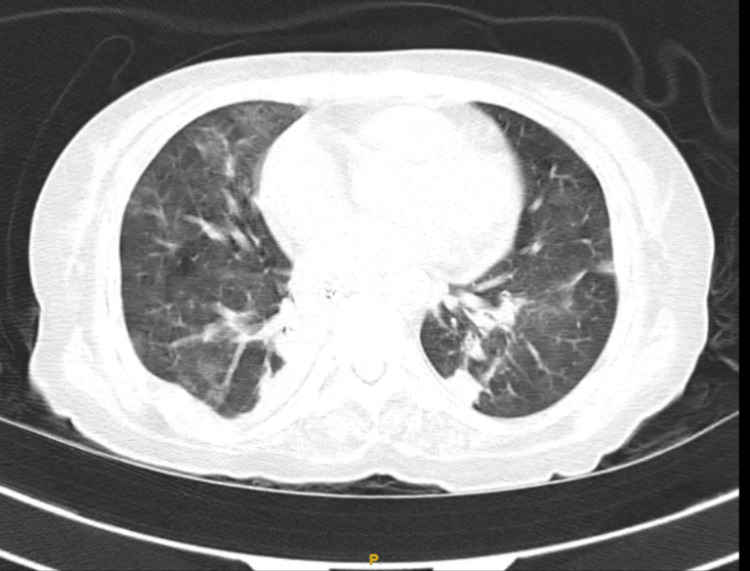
HRCT thorax showing bilateral patchy consolidation with cardiomegaly and bilateral diffuse ground glass opacity suggestive of pulmonary edema HRCT, high-resolution computed tomography
